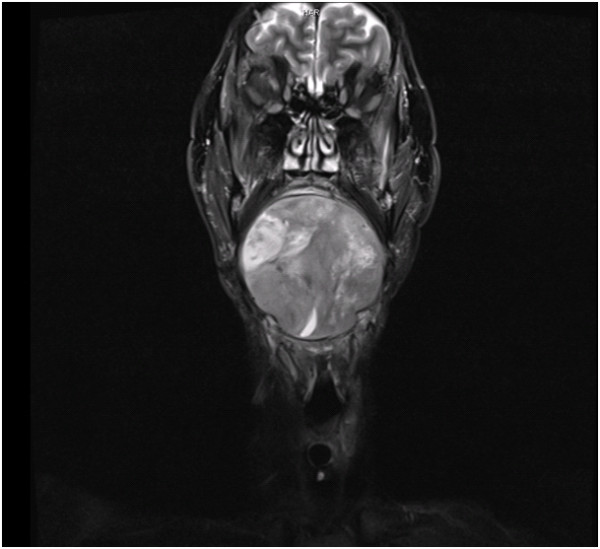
A preoperative magnetic resonance imaging scan in August 2011. The entire floor of the mouth, including the tongue, is filled by the tumor.
